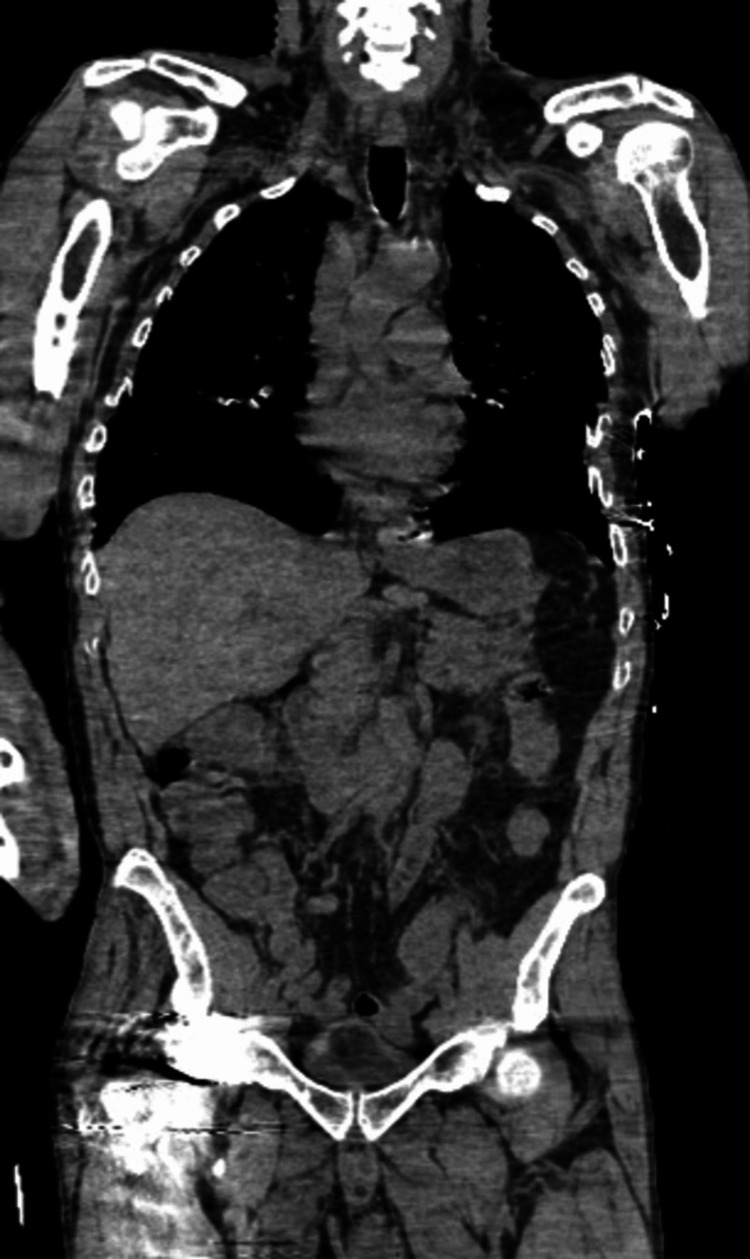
Normal chest/abdomen/pelvis computerized tomography (CT) scan
